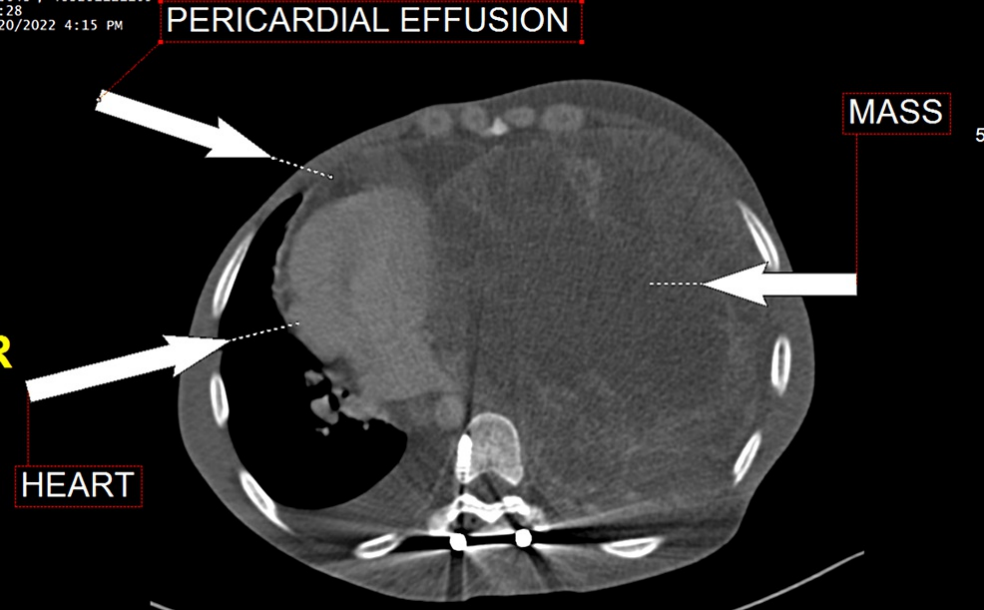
Contrast-enhanced chest CT revealing a large lobulated heterogeneous mediastinal mass. CT, computed tomography
